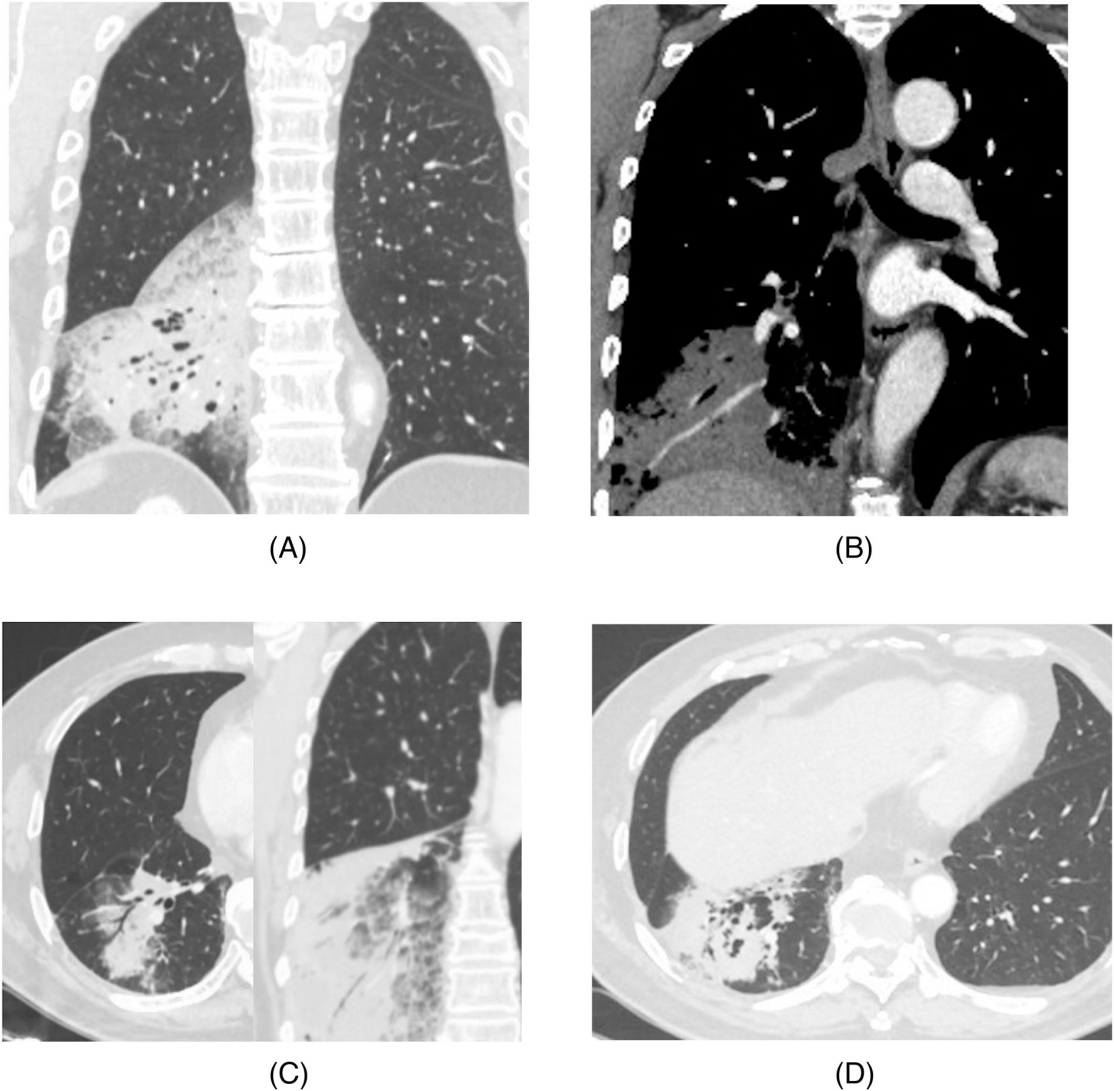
The CT features of large consolidative‐type invasive mucinous adenocarcinoma (IMA). (A) Halo sign refers to the ground glass shadow around the lesion, which results from the infiltration of a large amount of mucus and macrophages around the lesion area. (B) Angiogram sign refers to obvious enhancement of vascular shadow passes through the low‐density consolidation image on the enhanced CT. (C) Air bronchus and dead branch sign mean that the bronchus shadow can be seen in the consolidation area. The smooth bronchus is called the “air bronchus,” and the twisted and invaded bronchus is called “dead branch sign.” (D) Vacuole sign refers to the gas‐containing part of the lung with a diameter usually <5 mm. A cavity is produced by the expulsion or drainage of a necrotic part of the lesion via the bronchial tree with a diameter usually >5 mm.
